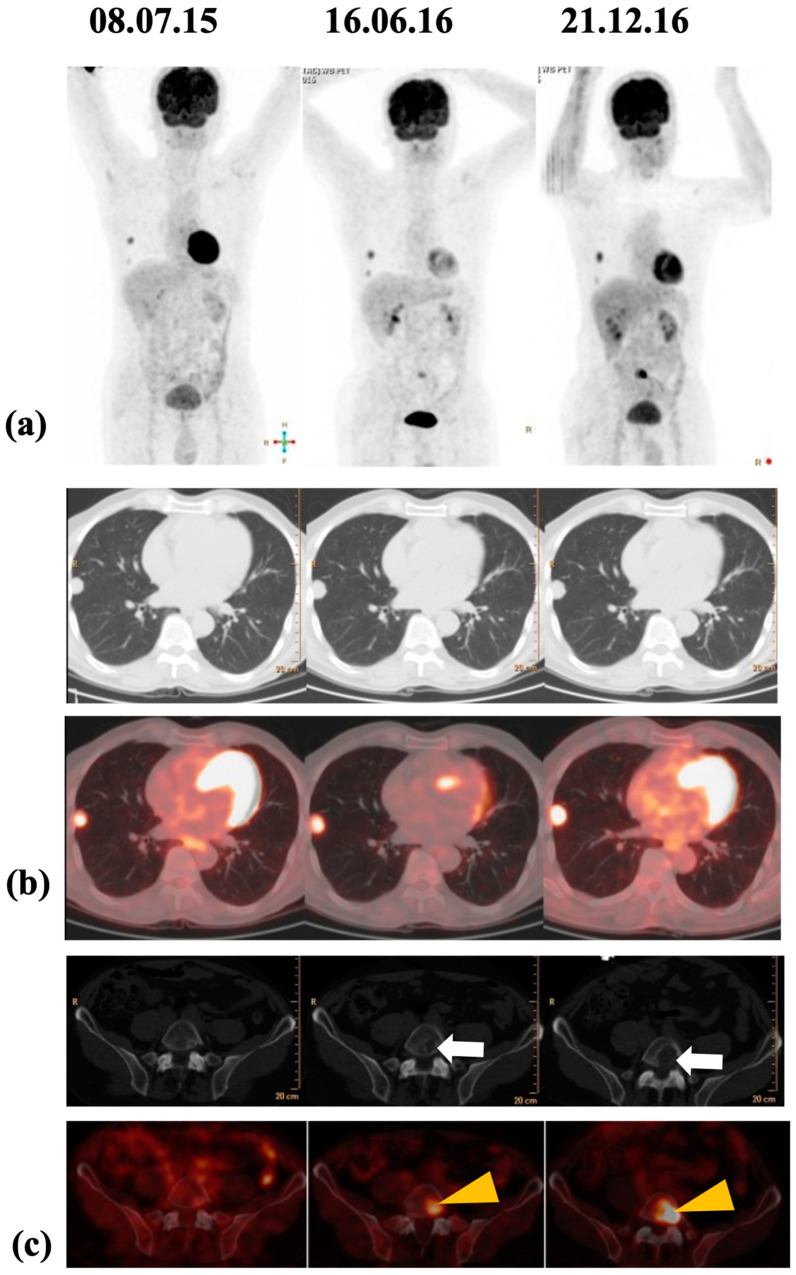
(a–c). Comparative ^18^F-fluorodeoxyglucose positron emission tomography/CT (FDG-PET/CT) maximum intensity projection (MIP) images (a), transaxial CT and fused PET-CT images of the lung lesions (b), and transaxial CT and fused PET-CT images of the L5 vertebral lesion (c) undertaken at 3 different time points of the disease course. While the bone metastasis is not a criterion in the standard RECIST criteria, the progressive disease was clearly evident in increasing indices on FDG-PET (arrowheadss) and osteolysis on CT (arrows). Reproduced with permission from: [92] 2017, Springer-Verlag Berlin Heidelberg.
